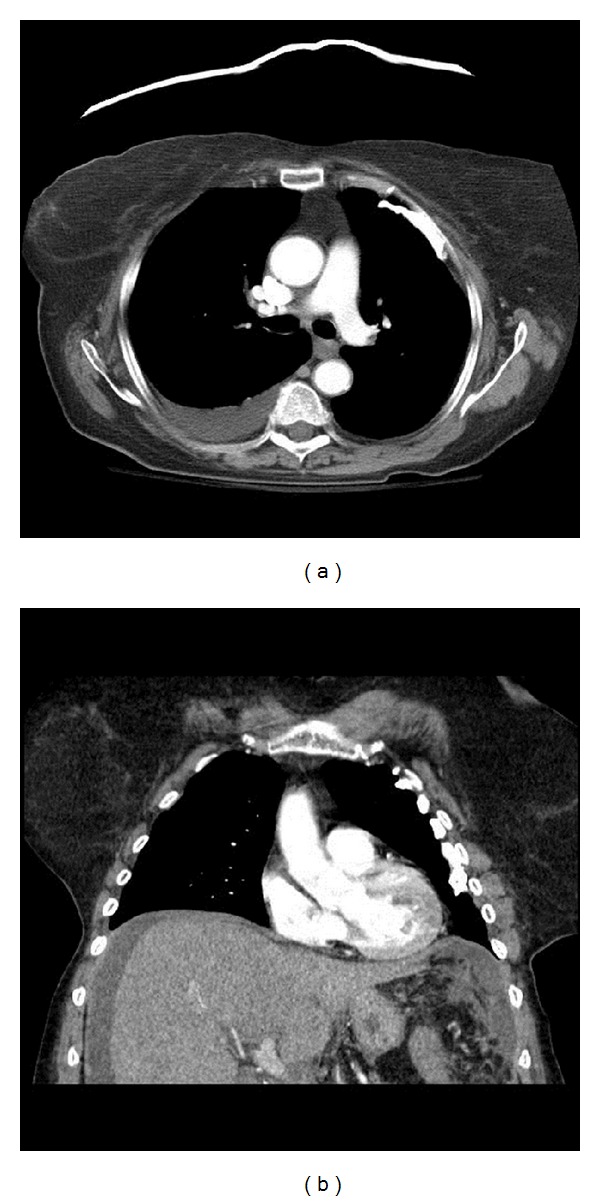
CT of the chest shows right-sided pleural effusion (a) and calcified pleural plaques in the left hemithorax (b).
